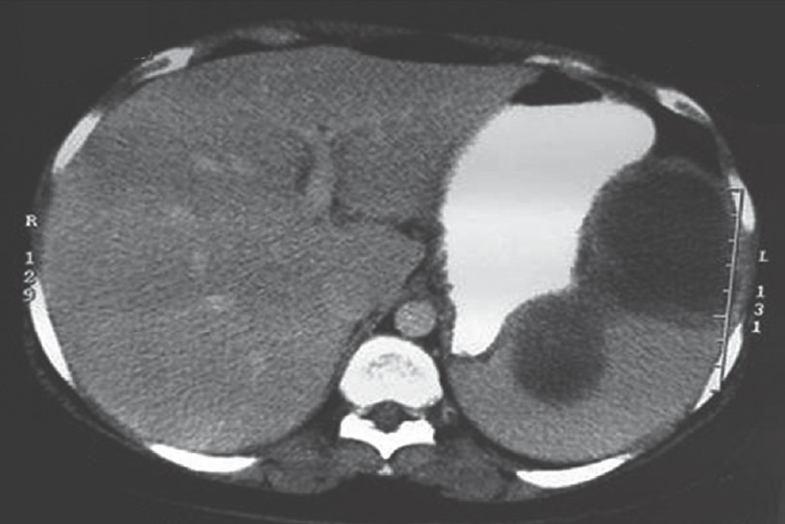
CT scan showing a large, multilocular splenic abscess
